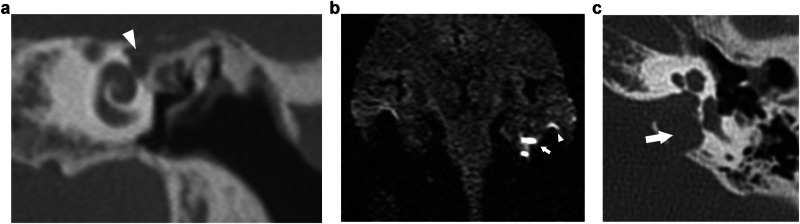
Fifty-five-year-old male with long-standing left-sided deafness, now presenting with a sudden onset of facial nerve paralysis. Supralabyrinthine (Class I) congenital petrous bone cholesteatoma (CPBC) in the left temporal bone. Coronal (a) and axial (b) computed tomography (CT) images of the left temporal bone show a well-circumscribed lytic lesion in the supralabyrinthine region with involvement of the geniculate ganglion (arrowhead in a), posterior extension medial to the vestibulum and dehiscent internal auditory canal (IAC) (arrow in b). There is high signal intensity in the medial petrous bone on EP DWI (b1000) (c) partially corresponding to the known cholesteatoma (arrow in c). There is a distorted aspect of the cholesteatoma making it less conspicuous. EP DWI is more susceptible to artifacts, mainly between the temporal bone and brain tissue (arrowhead in c), and has a lower in-plane resolution compared to non-EPI (compared to the DWI in Fig. 6)
